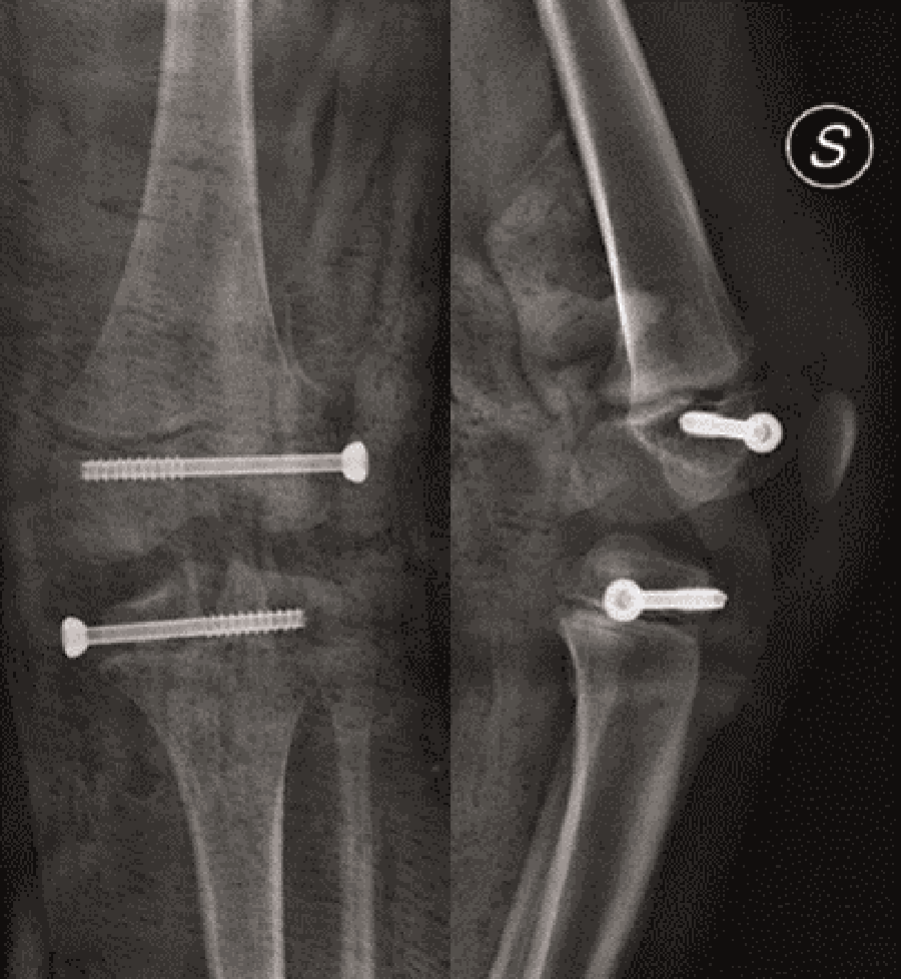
Postoperative x-ray control after reduction and synthesis of left knee growth plate fractures.
